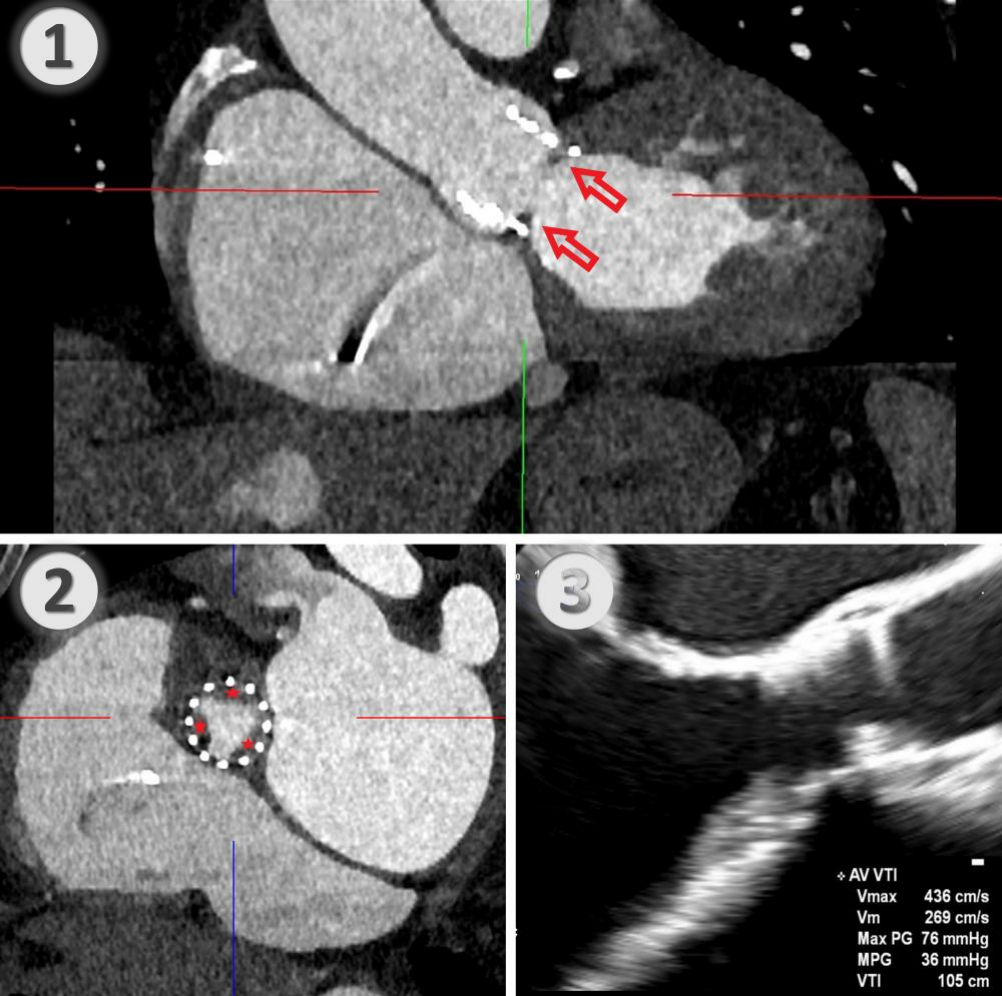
(*1*) Sagittal view of the prosthetic valve in contrast enhanced computed tomography. Arrowheads: pannus formation beneath valve leaflets. (*2*) Short axis view of prosthetic valve with pannus formation at the level of prosthetic valve cusps. Asterisks: pannus beneath all three valve leaflets. (*3*) Transoesophageal echocardiography images of the prosthetic valve in long axis view with transprosthetic gradients.
